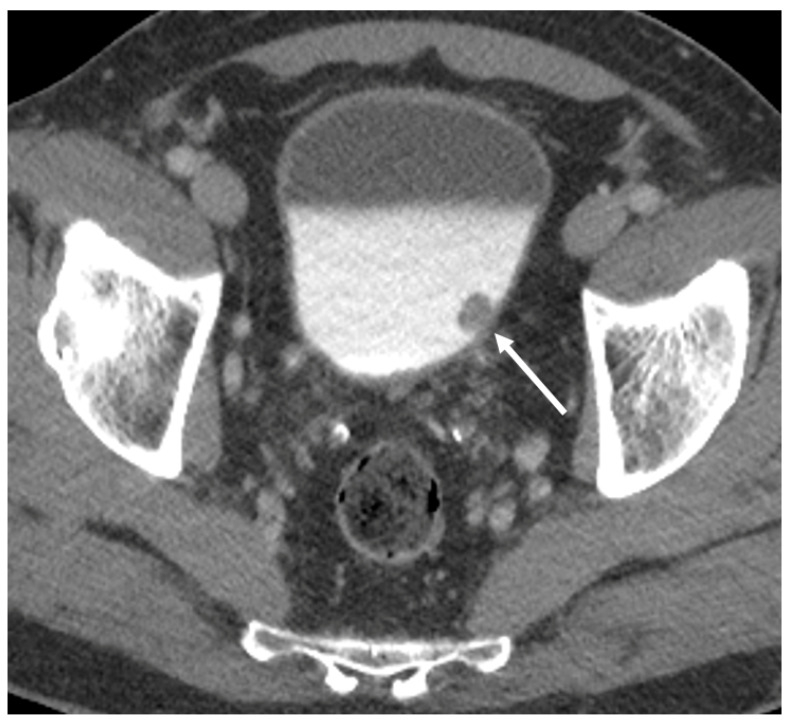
Axial CT images from the excretory phase of a CT urogram performed for hematuria demonstrating a polypoid filling defect arising near the left ureteral orifice (arrow), highly suspicious for bladder cancer.
